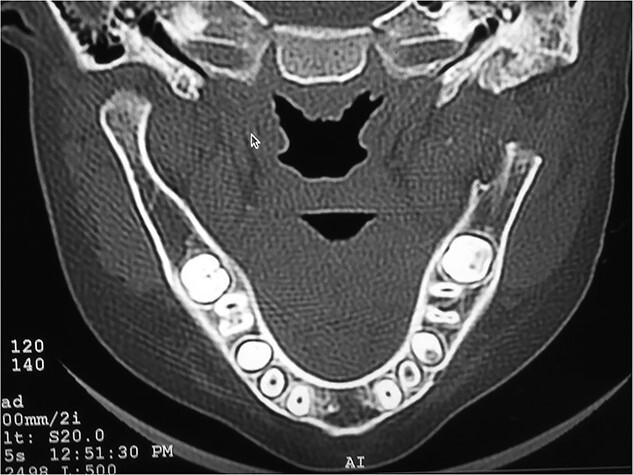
Post-operative coronal section CT image.
